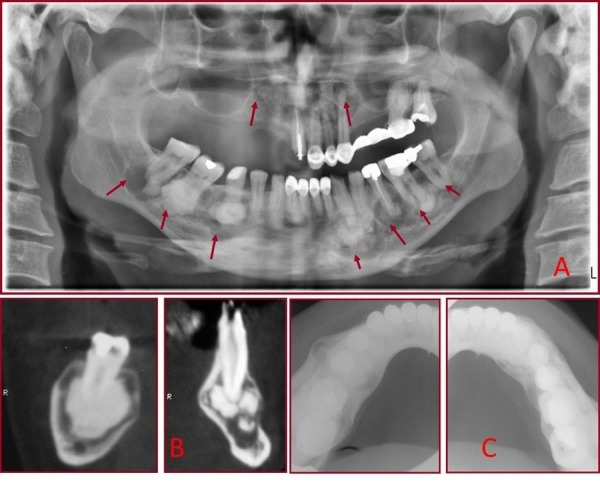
A. Panoramic radiograph showing multiple mostly dense mixed radiodense/radiolucent lesions closely associated with the roots of maxillary and mandibular teeth. Note that the epicenter of the lesions is above the inferior alveolar canal; B. Vertical reconstruction at level of the lower right second premolar and first molar region; C. Occlusal radiograph showing slight buccal expansion at the molar region in the right and left side of the mandible.
